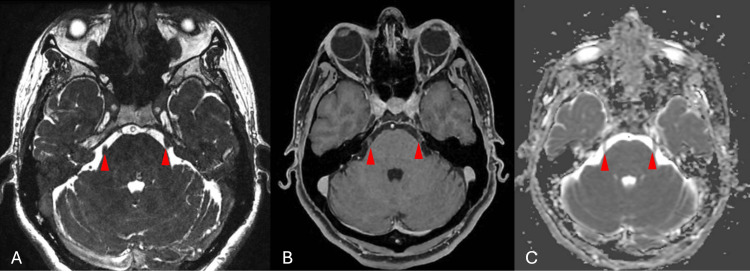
Preoperative brain MRI. (A) Axial T2-weighted FIESTA image showing no vascular compression of the root entry of the trigeminal nerves (arrowheads). (B) Axial contrast-enhanced T1 image without evidence of enhancing lesion. (C) ADC map demonstrating no restricted diffusion. The arrowheads indicate the trigeminal nerves bilaterally at the cisternal segment, demonstrating the absence of neurovascular compression. FIESTA: Fast Imaging Employing Steady-state Acquisition; ADC: apparent diffusion coefficient
